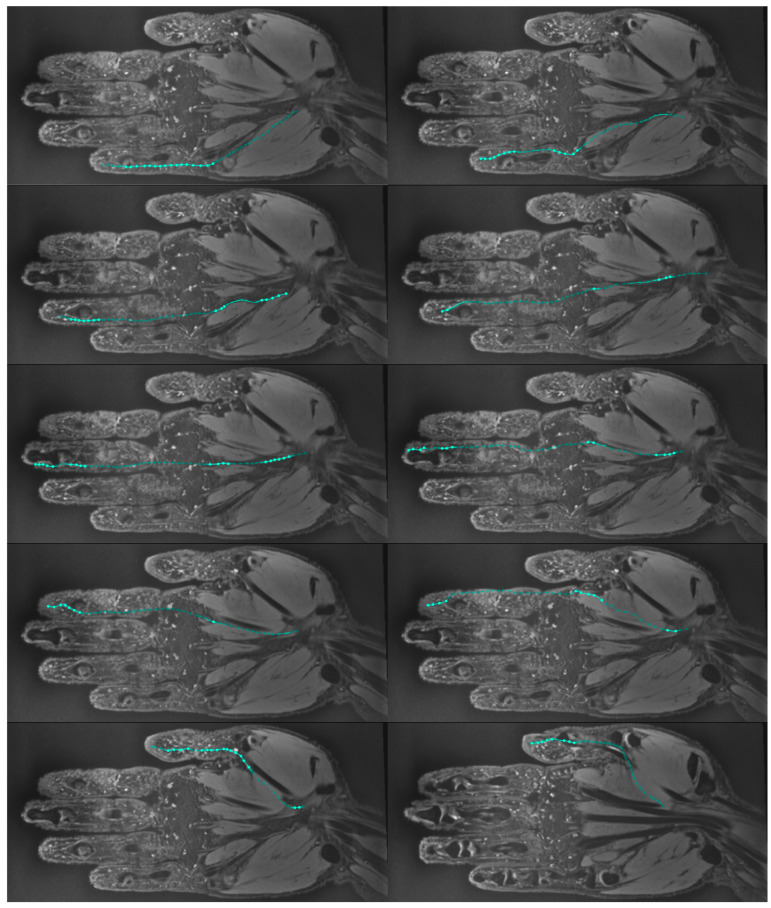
T2 DESS 3D MRI images of volunteer 3’s left hand in the coronal plane with palmar nerve paths, manually tracked with the 3D curved planar reformation function, as provided by OSirix (see blue lines and dotted landmarks).
